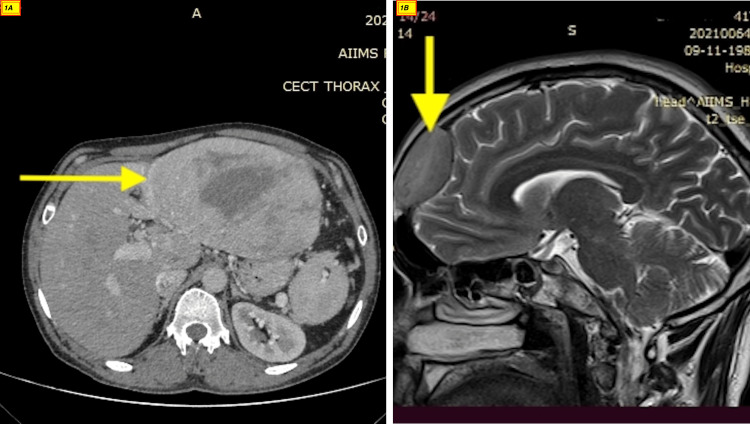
CT abdomen and MRI brain of the patient. The image shows a large lobulated altered signal intensity lesion measuring 122x76x148 mm in the epigastric region with non-separate visualization of pancreas and left liver lobe (A). An active lesion in the right frontal region of the brain with lytic changes in the frontal bone (B).
